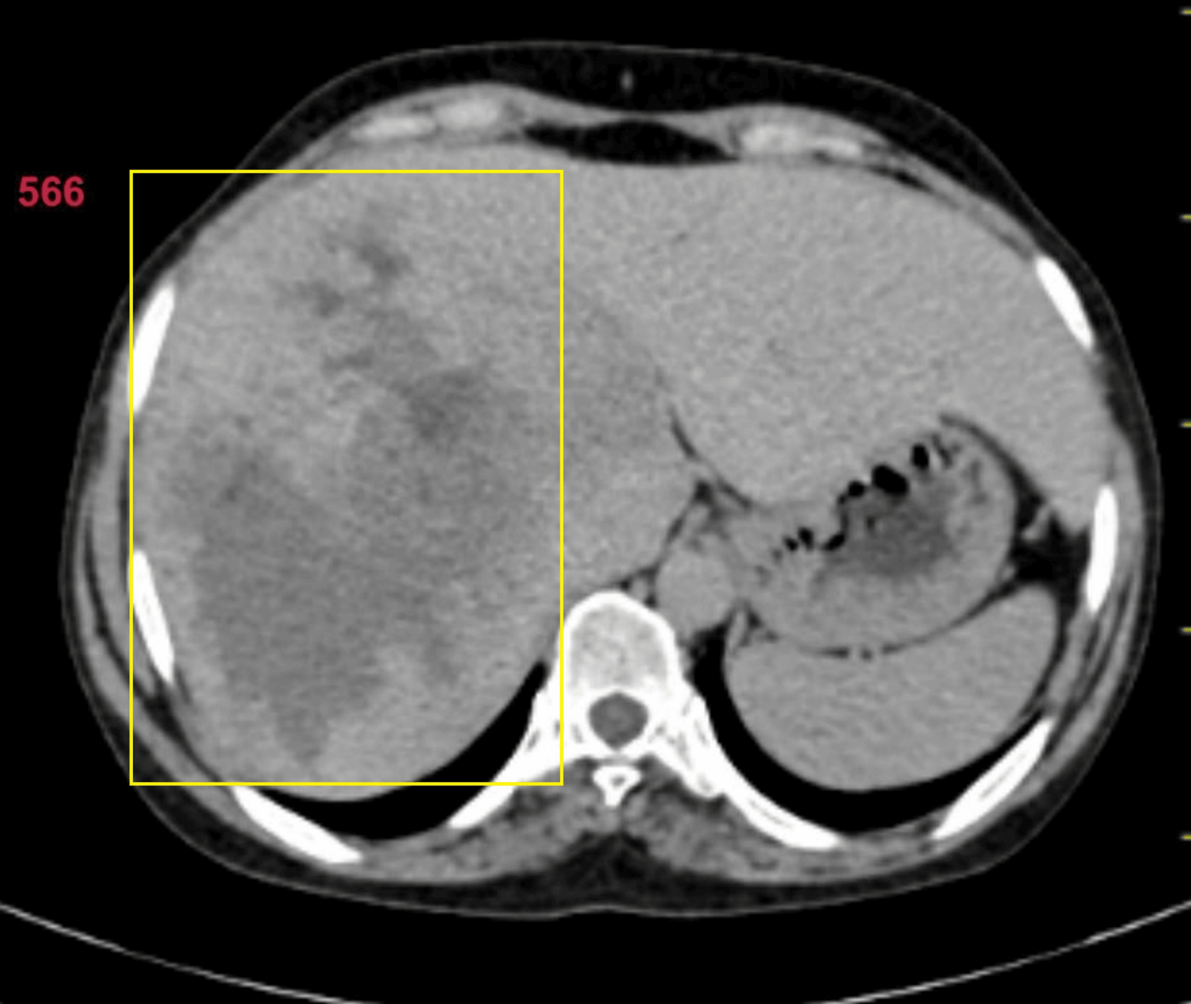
CECT of the pelvis showing hemangioma in the liver CECT: contrast-enhanced computed tomography
